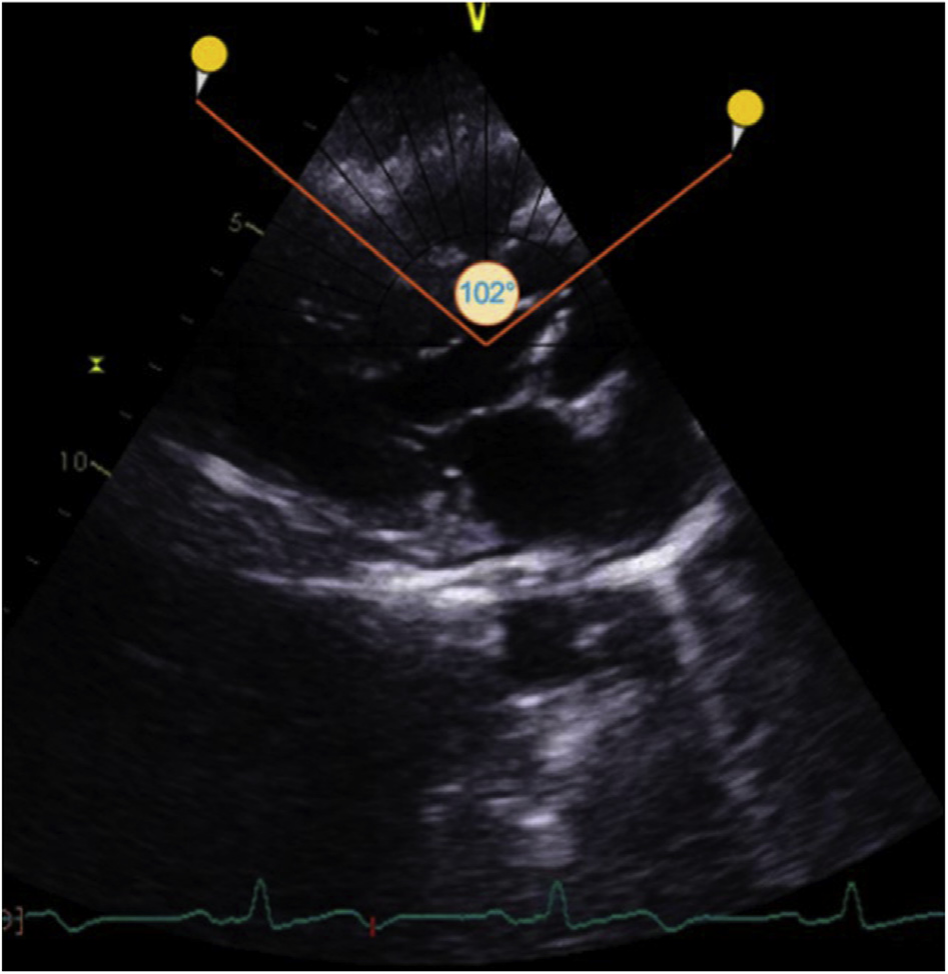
Two-dimensional TTE parasternal long-axis view showing an aortoseptal angle of 102° measured using an online protractor.
